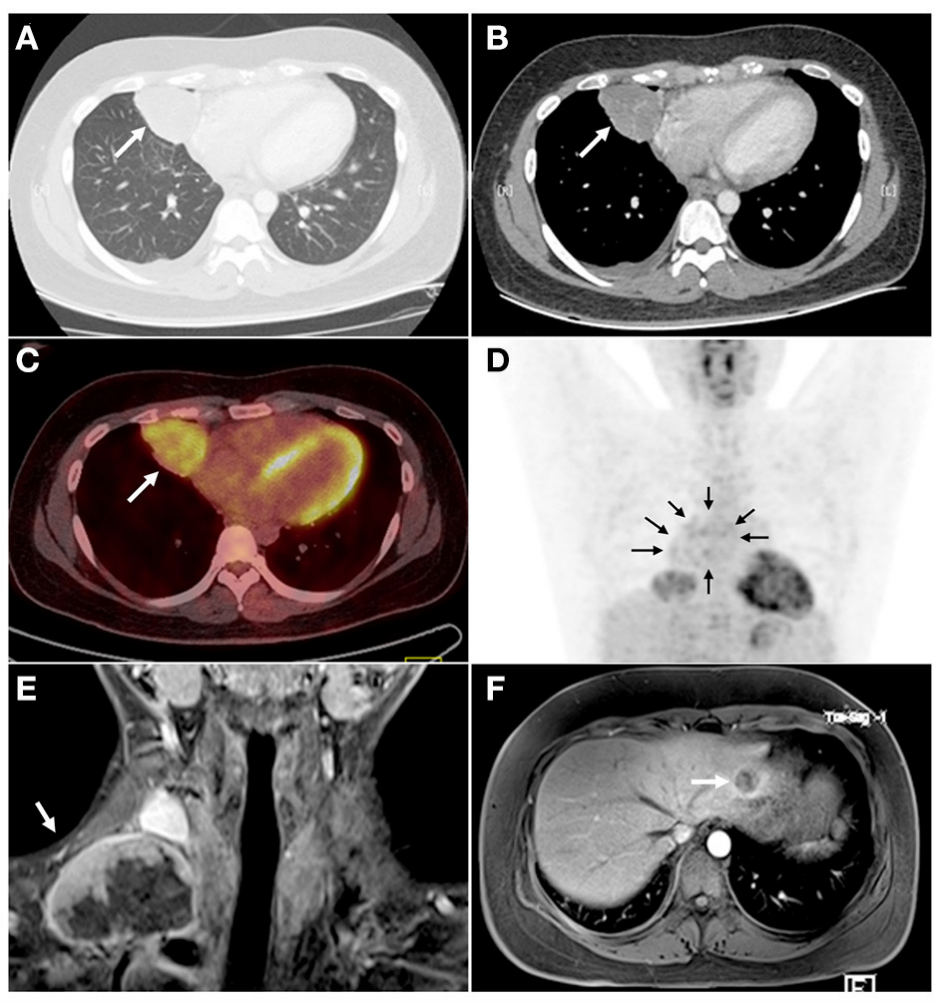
CT, PET, and MRI scans showed a primary lesion in the right lung and metastatic tumors in mediastinum, right neck, and right liver. (A) The lung window of CT examination showed a round-like mass (arrow) in the medial segment of the right middle lobe with a smooth margin. (B) The lesions (arrow) were slightly enhanced, and the tortuous small vessel shadow was seen inside. (C,D) PET examination showed that the radioactivity uptake of the right pulmonary pericardial lesions was increased (C, arrow), and the suspected annular radioactivity uptake in the mediastinum (D, arrows) was increased. No obvious abnormal radioactivity uptake was seen in other organs. (E) The neck MRI showed metastatic tumors (arrow) in the right neck after 5 months of the operation (thoracoscopic assisted resection of the right middle lung and systemic lymphadenectomy). The lesion was uneven enhancement and had abundant necrotic areas. (F) The abdomen MRI showed a lesion in the left lateral lobe of the liver (arrow). CT, chest enhanced computed tomography; PET, positron-emission tomography; MRI, magnetic resonance imaging.
